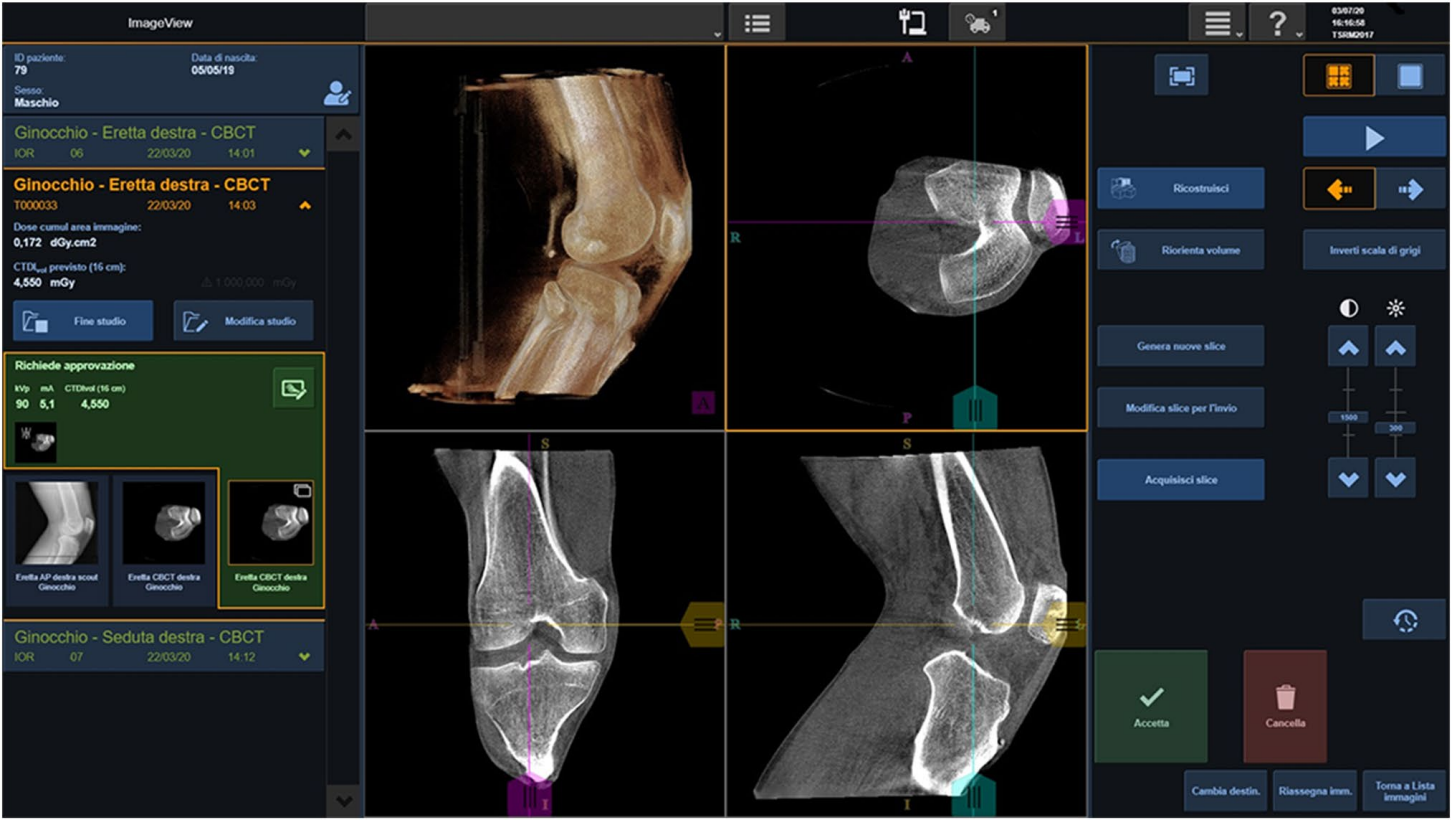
Screenshot from CBCT device with of the three anatomical plus the 3D rendering views
